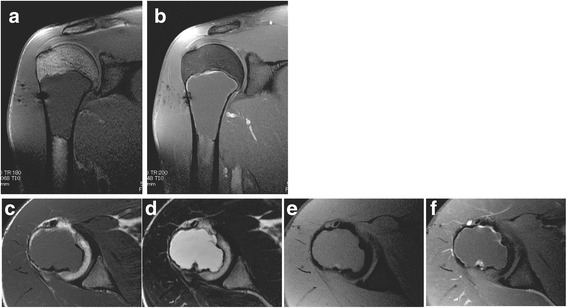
a–f MRI scans of the right shoulder at 3 years after initial presentation. T1-weighted coronal (a), T1-weighted transverse (c), and with gadolinium contrast (f) images of the proximal humeral lesion showing a homogenous low signal intensity. T2-weighted coronal (b), transverse (d), and fat-suppressed (e) images of the proximal humeral lesion showing a homogenous high signal intensity
